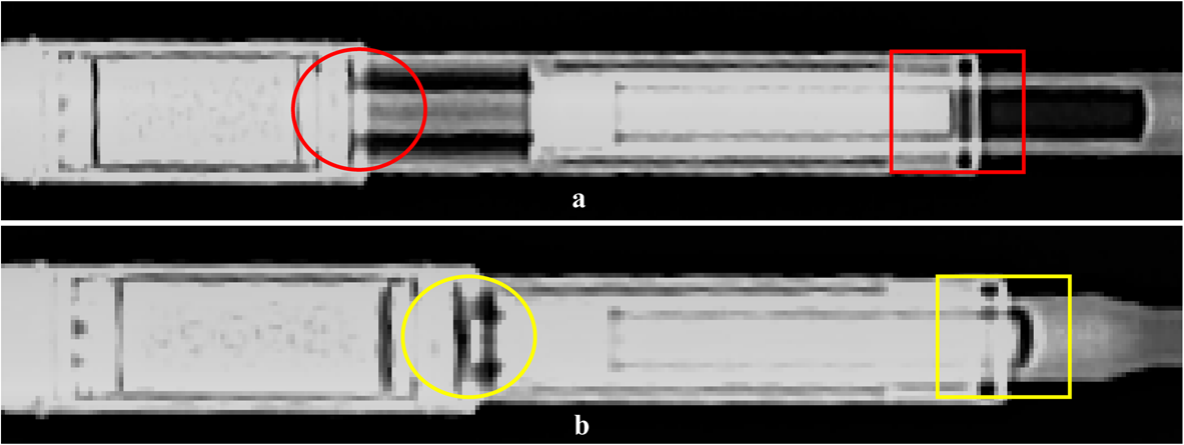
Plain radiographs of two MAGEC rods with intact (a) and damaged (b) internal mechanism. In the first rod (a), the connection of internal mechanism was intact (*circle*) and the internal driving screw was within the actuator case (*box*). In the second rod (b), the internal mechanism was disconnected (*circle*) and the internal driving screw was beyond the actuator case (*box*)
